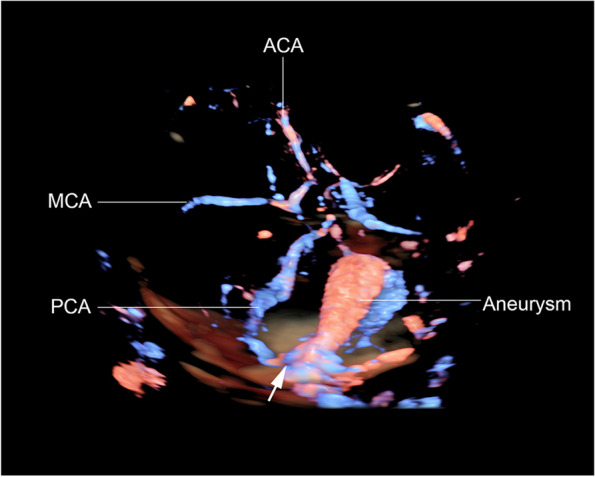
Rendered 3D-color flow ultrasound of the fetal brain at 36 weeks of gestation shows course of posterior cerebral artery (PCA) feeding the aneurysm from lateral wall of parieto-temporal hemisphere (arrow: indicating originating point of flow jet feeding the aneurysm). (ACA: anterior cerebral artery; MCA: middle cerebral artery)
